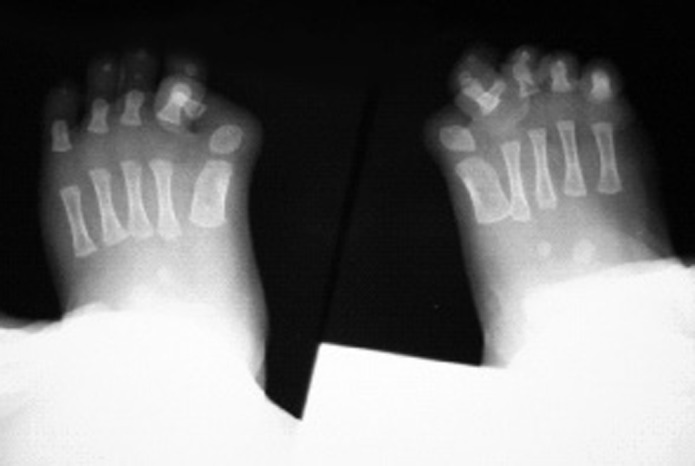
PA radiography of the feet shows bilateral hallux valgus congenital malformation as a hallmark of FOP
